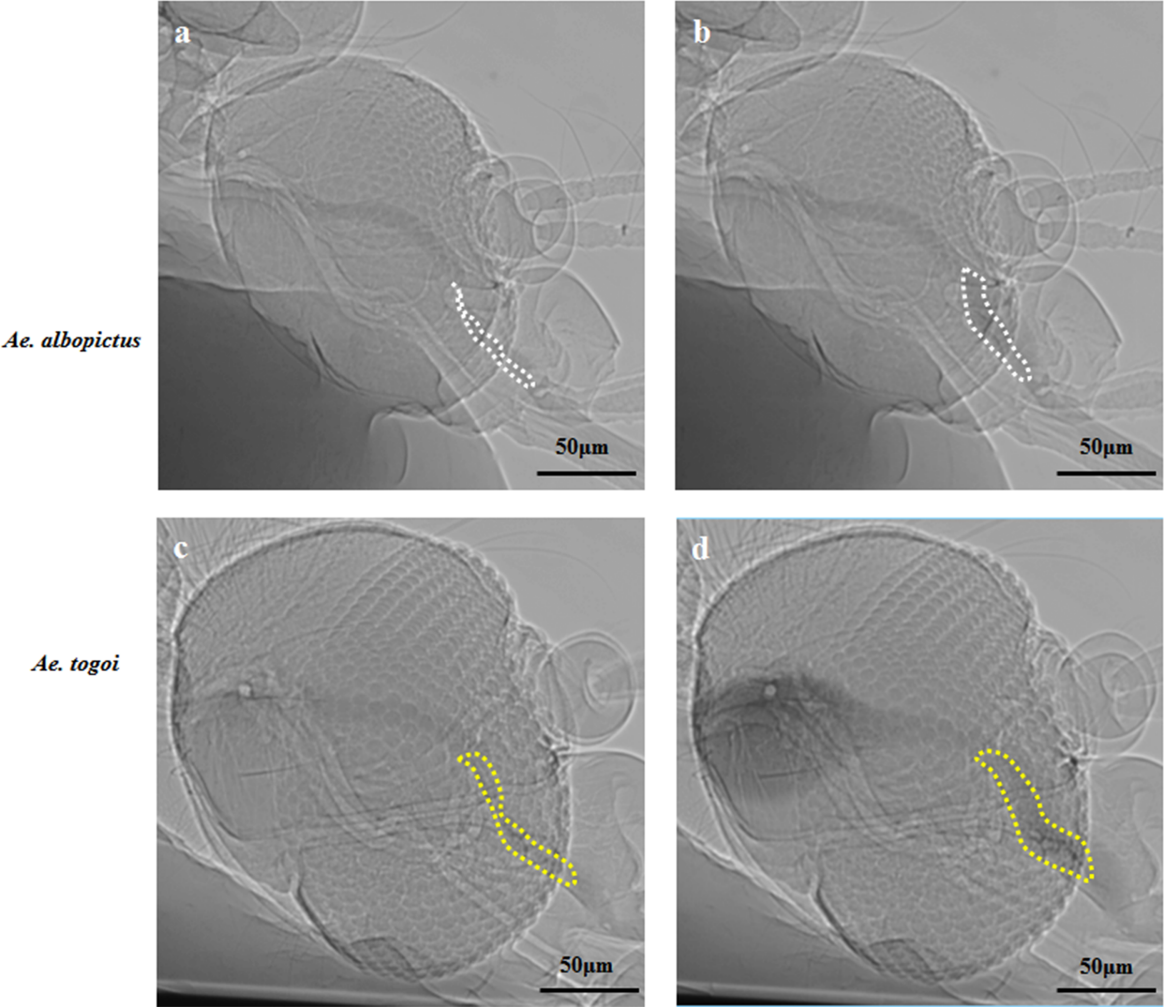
Variations in the CP cross-sectional area. The minimum CP area of *Ae*. *albopictus* (a) and *Ae*. *togoi* (c). The maximum CP area of *Ae*. *albopictus* (b) and *Ae*. *togoi* (d). The cross-sectional CP area was estimated from the X-ray images captured by the synchrotron X-ray micro-imaging technique. A diluted iodine solution was used as the feeding fluid to enhance image contrast.
